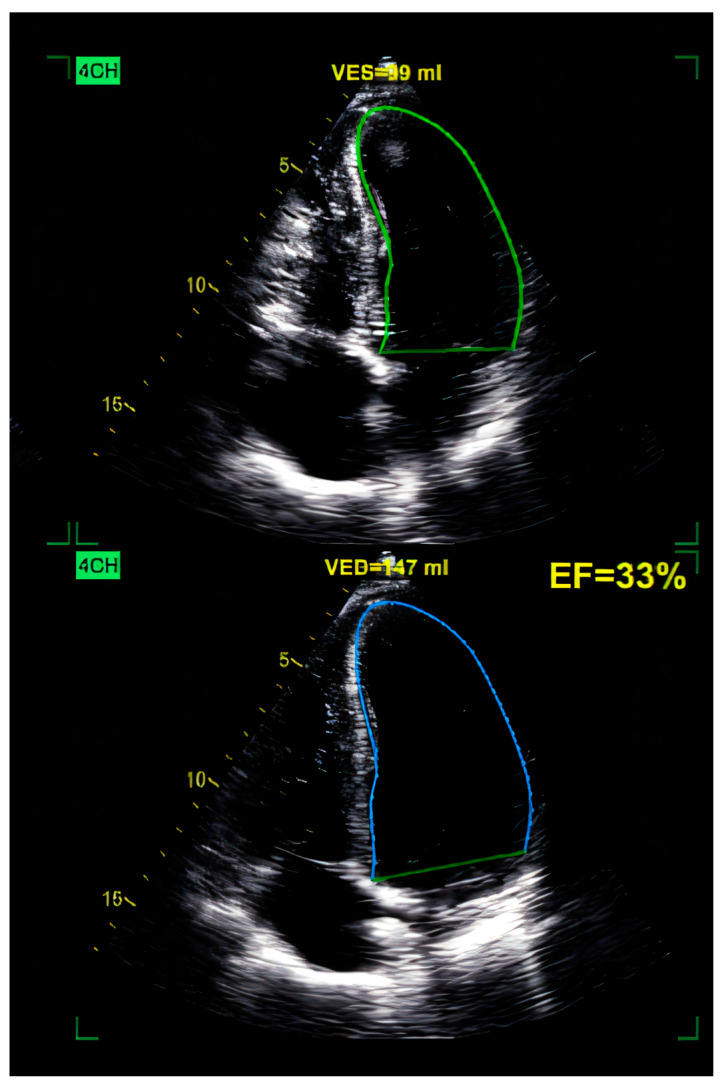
Two-dimensional TTE (transthoracic echocardiography) of the LV (left ventricle), revealing a dilated LV of 147 mL, with globally reduced EF of 33% and an apical thrombus (upper green area) in a patient with anterior MI. 4CH = apical 4-chamber view.
